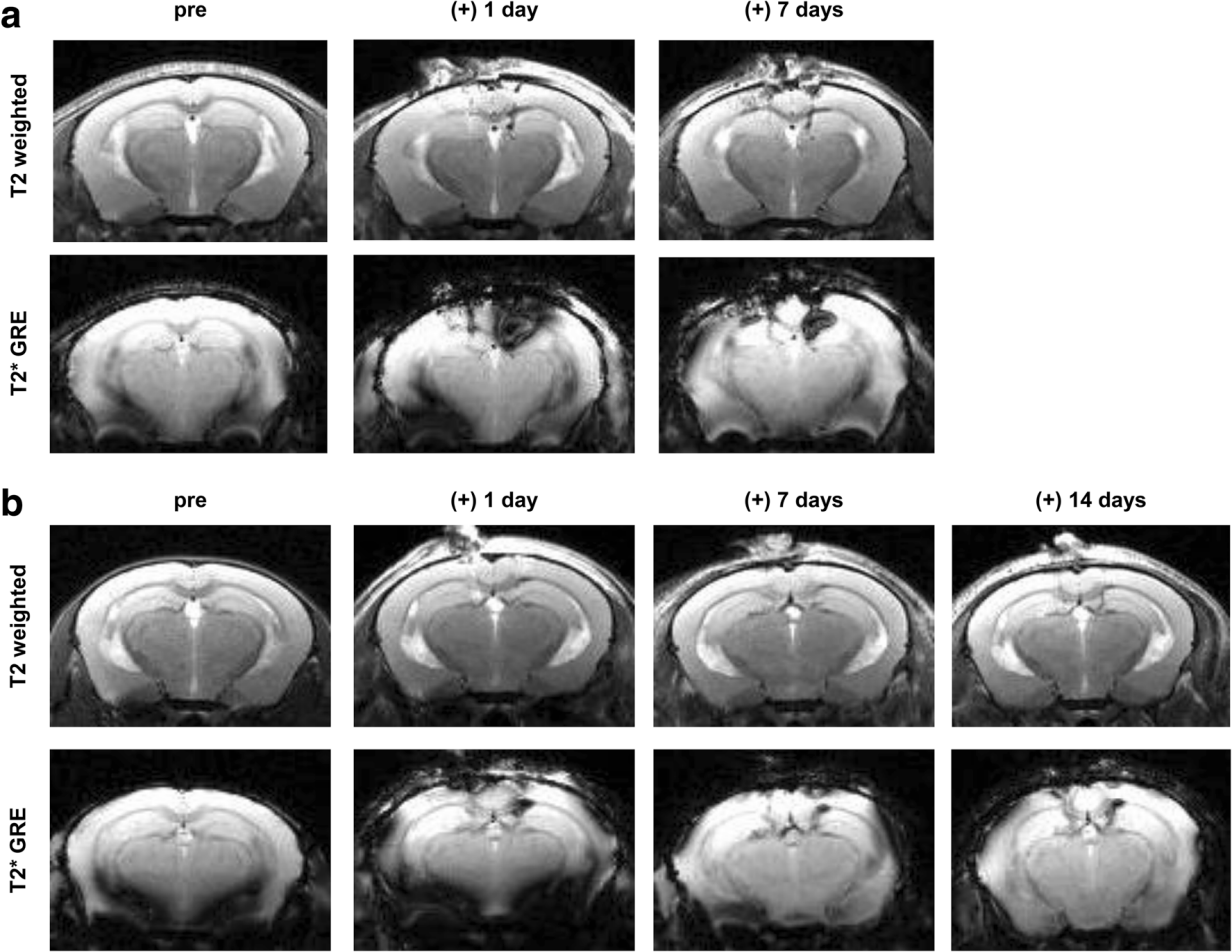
Hypointense Signals Consistently Visualized at Extended Time Points. MR images acquired up to (a) 7 and (b) 14 days post transplantation. Similar to early time points, hypointense signals were observed in the right hippocampus where the ferumoxtyol-labeled hUCB-MSCs were transplanted. A gradual decrease in the size of the signal intensity was apparent at post 7 days while striking differences were not noted between post 7 and 14 days
